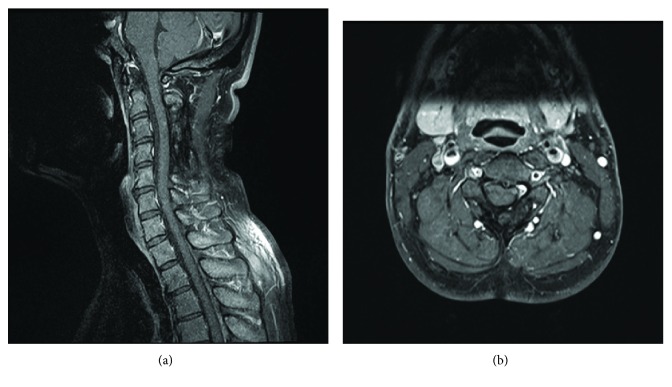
(a, b) MRI of the cervical spine performed four years after the surgery showed no recurrence, and the dural tail sign had disappeared.
